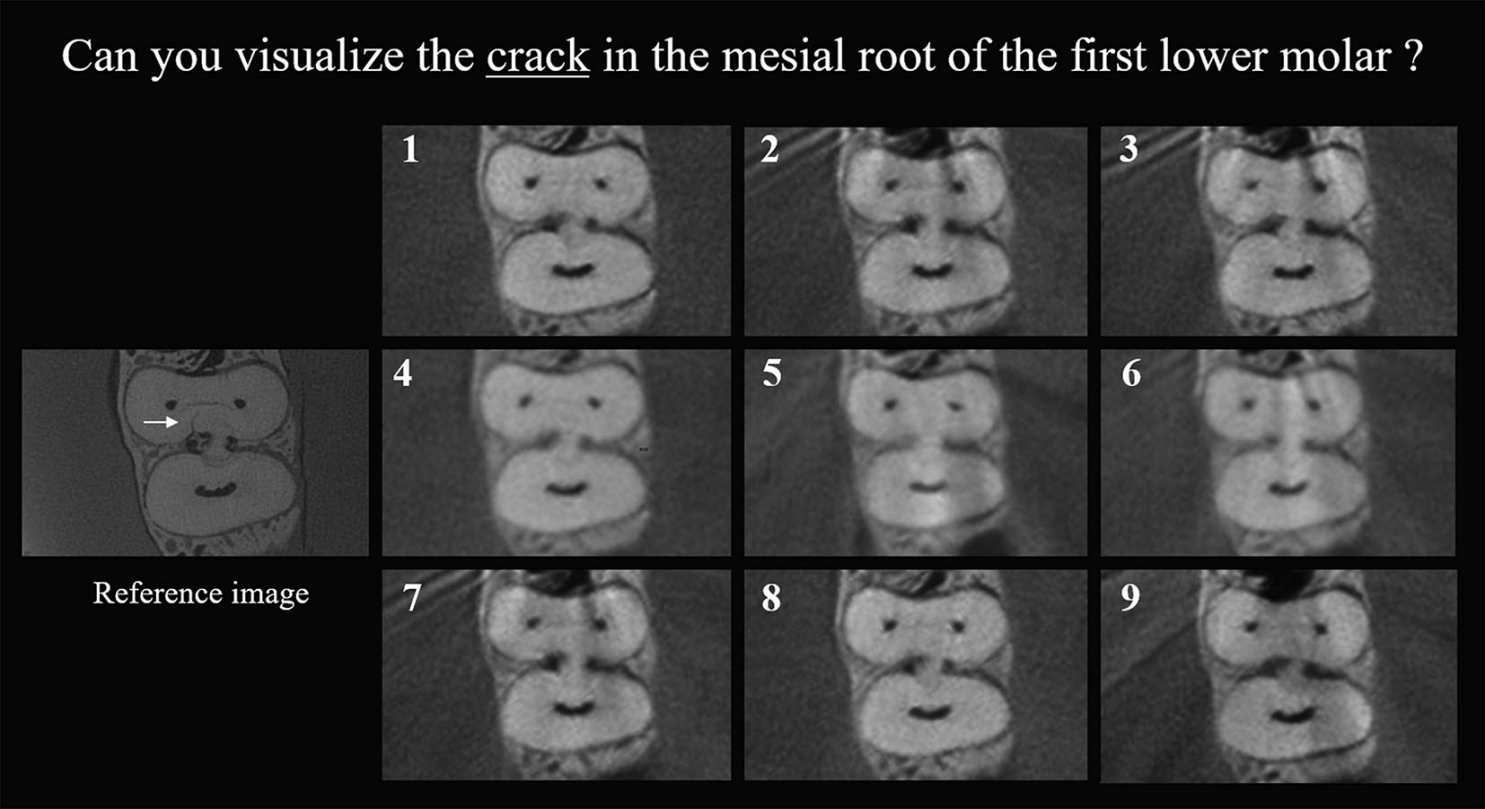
Set of images used for crack assessment containing a reference image from industrial CT and numbered images of X1 CBCT device for all conditions and scanning protocols.
